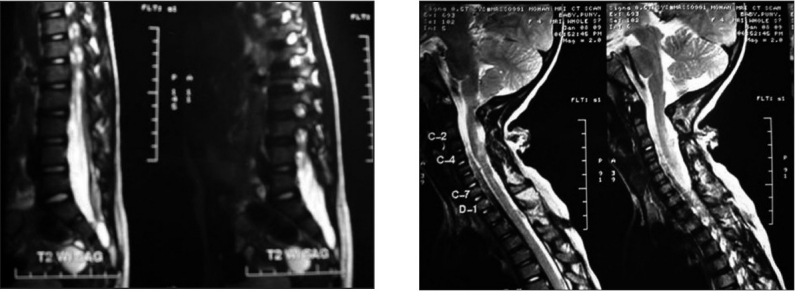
Magnetic resonance imaging (MRI) of the spine showing tethering of the cord with lipomatous filum terminale and cervicothoracic MRI showing swelling at the C2–C4 level and sinus at the D4 level
